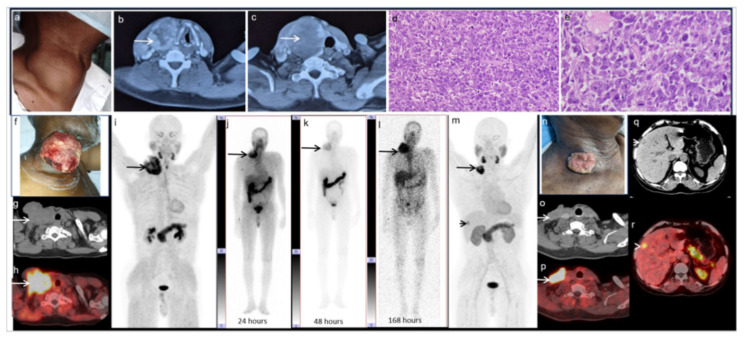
A 56-year-old man with a long-standing anterior neck mass presented with rapid thyroid enlargement over 1 month, associated with dyspea and dysphagia (a). Contrast-enhanced CT revealed a large heterogeneously enhancing right thyroid lesion with marked tracheal displacement (b,c). Highly cellular tumor with pleomorphic polygonal cells, brisk mitotic activity, atypical mitoses, and necrosis (d,e). He received EBRT (external beam radiotherapy) of 30 Gy in 15 fractions to the neck; however, during EBRT the mass rapidly grew in size at the surgical site (f). [^68^Ga]Ga-DOTA.SA.FAPi PET/CT demonstrated intense tracer uptake in the recurrent neck mass on CT (g), fused PET/CT (h), and MIP images (i, arrows). The posttherapy scans (j–l, arrows) revealed avid and sufficiently prolonged tumor retention, even at 168-h posttreatment (l) scan. Follow-up [^68^Ga]Ga-DOTA.SA.FAPi PET/CT demonstrated significant reduction in the recurrent neck mass on MIP (m), clinical examination (n), CT (o), and fused PET/CT (p); a new hepatic lesion in segment VIII (m,q,r, arrowheads) [106].
